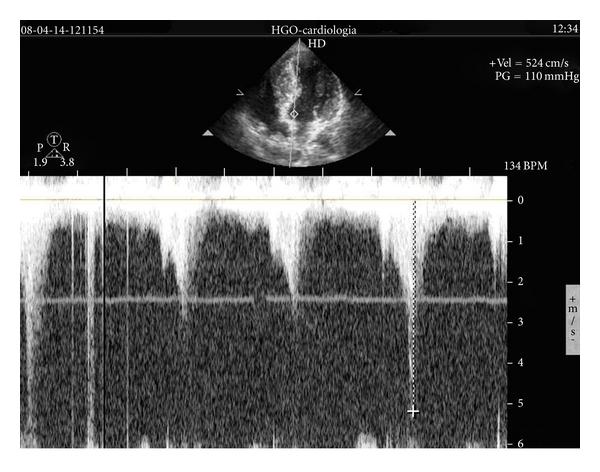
Flow evaluated with continuous wave Doppler after exercise demonstrating the presence of LVOT obstruction.
